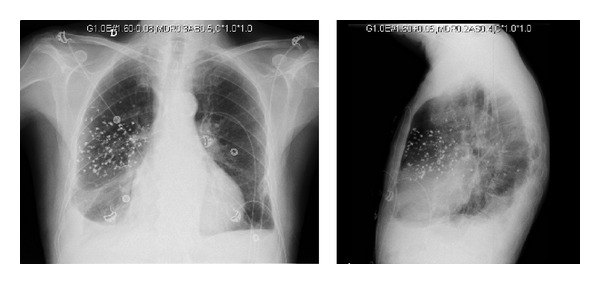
Chest radiograph in posteroanterior and lateral projections.
